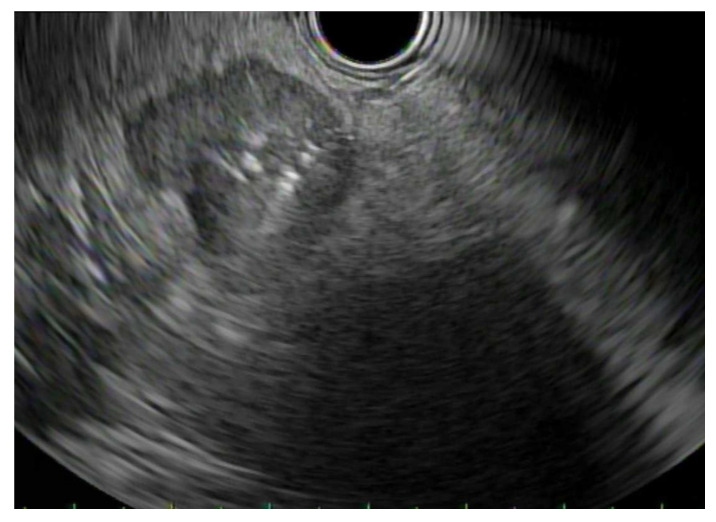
EUS-Radiofrequency Ablation (EUS-RFA): Endosonographic image of a pancreatic lesion being ablated by EUS-RFA.
